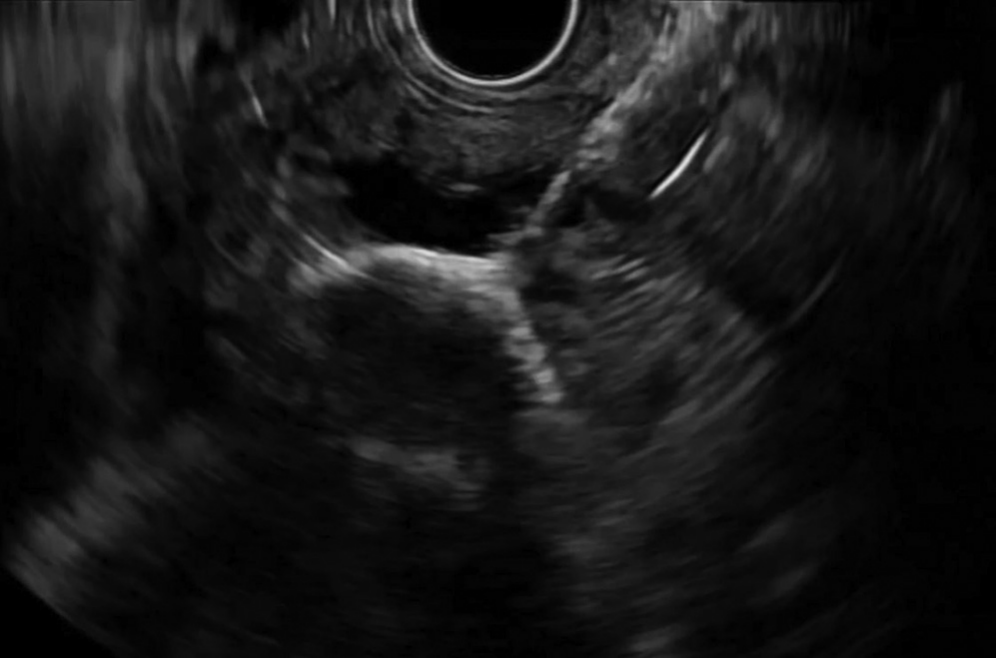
Endosonographic image showing distal flange of lumen-apposing metal stent deployed in the jejunal limb.
